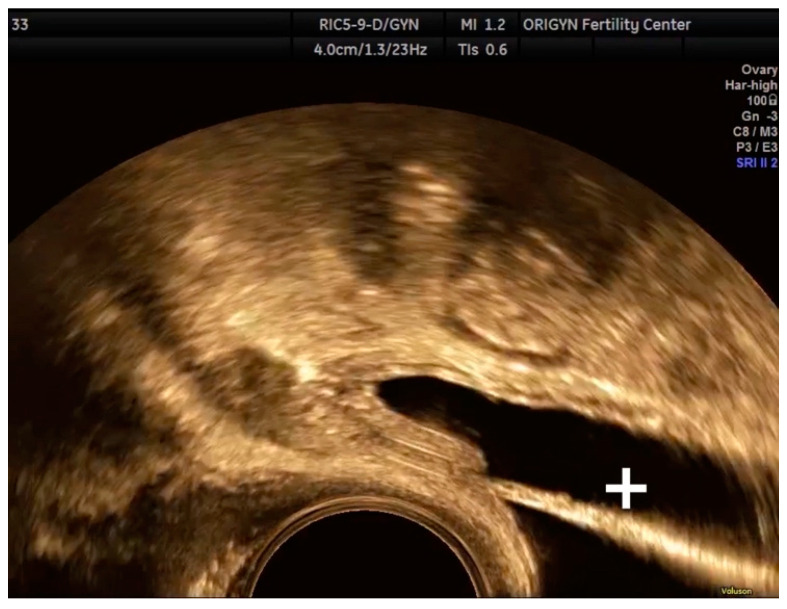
Transvaginal ultrasound of the bladder showing the ureter and the JJ stent in a normal position. (+ sign in the figure plays the role of a pointer to highlight a specific feature).
